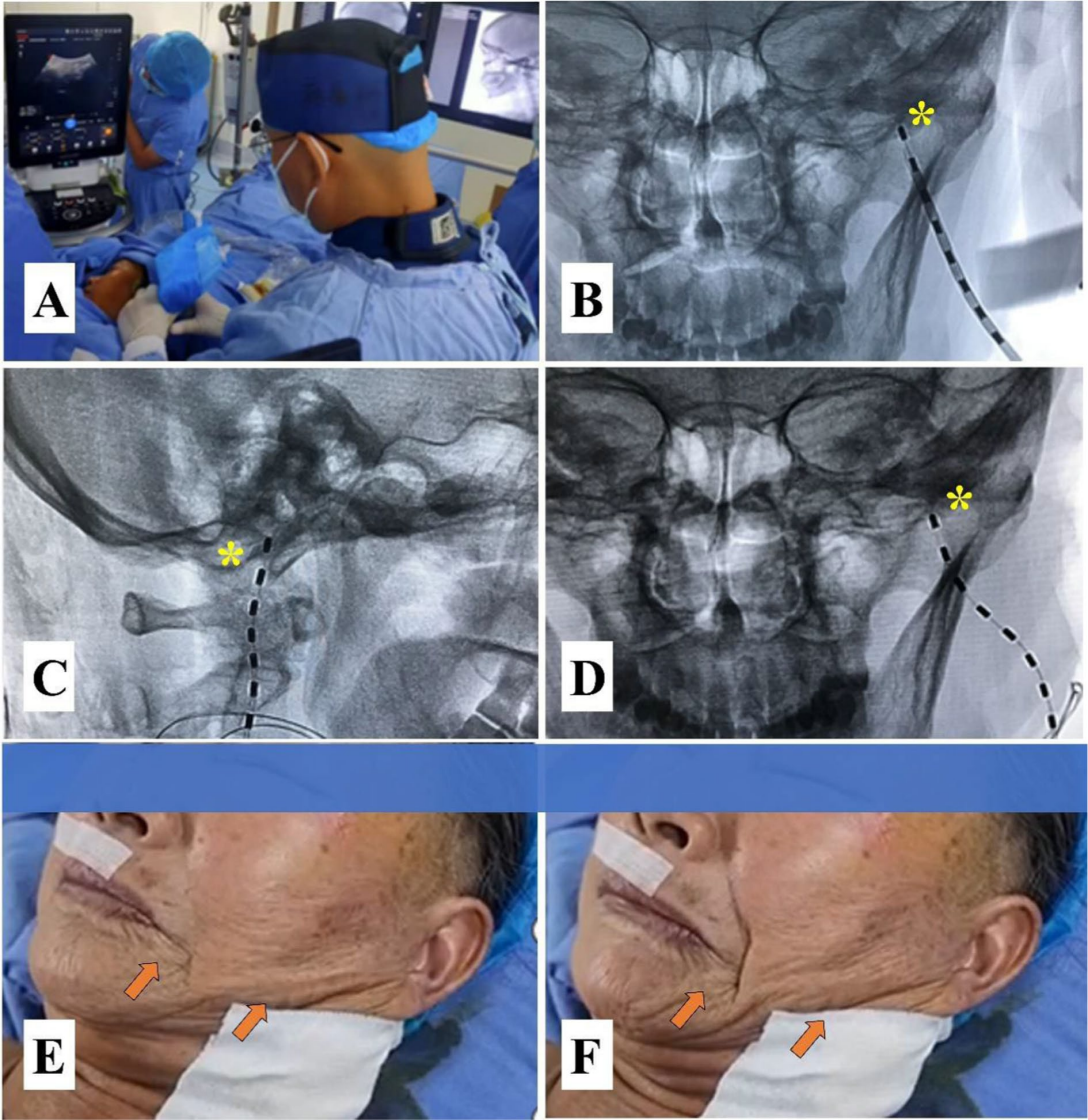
A pilot protocol for transcutaneous facial nerve stimulation (t-FNS). (A) Ultrasound-guided injection of 0.5% lidocaine for regional anesthesia and widening of the puncture route to avoid intravascular injection. (B) The lead was implanted medial to the MP using a manual 15° arcuate hollow needle in the anteroposterior view of the DSA. (C) The lead was again placed anterior to the MP in the lateral view of the DSA. (D) The anteroposterior view of the DSA confirmed that the lead was placed medial to the MP. (E) A slight contraction of the facial muscle was elicited by a test stimulation voltage of 0.3 V. (F) A significant contraction of the facial muscle was elicited by a stimulation voltage of 1 V. The yellow asterisk indicates the MP, and the orange arrow represents the contraction of the facial muscle.
